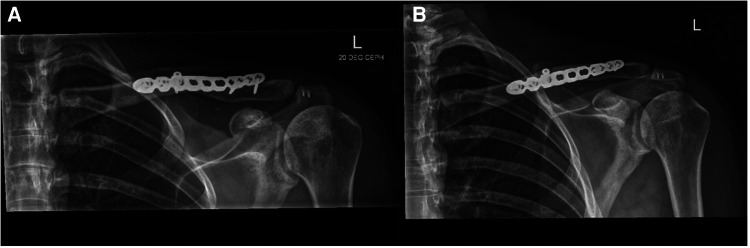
Radiographs demonstrating a united fracture and stable acromioclavicular joint post removal of hook plate.
